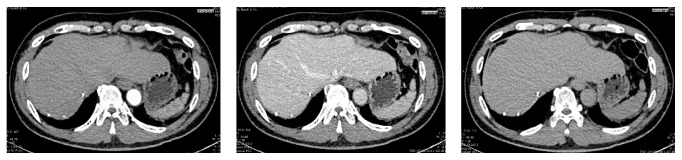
Arterial, venous, and delayed phase of Abdominal CT Scan 3 years after surgical resection (November 2016).
